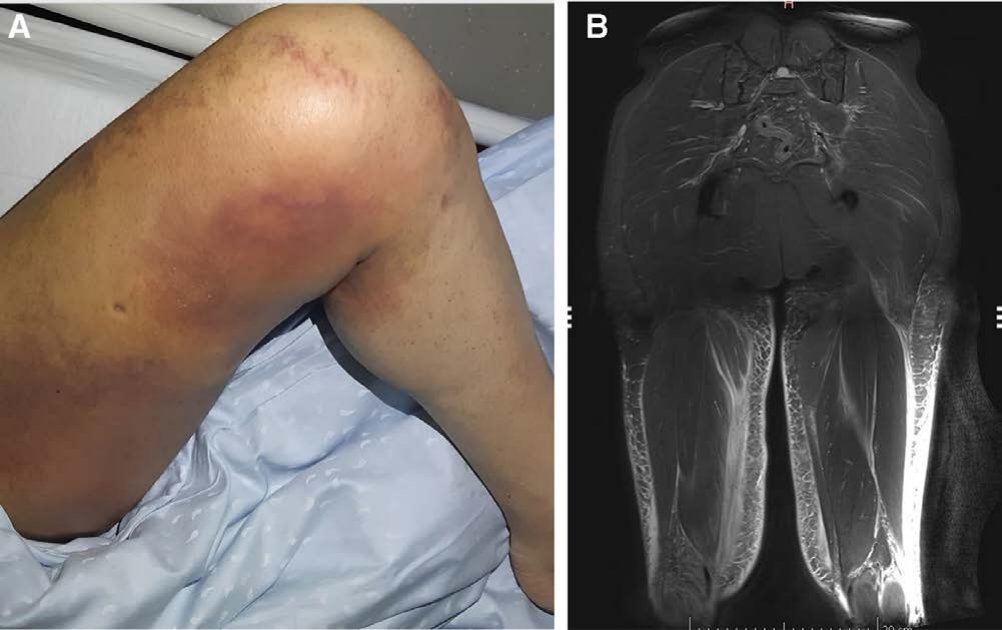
(A) Picture of the lower limbs showing both edema and hematomas. (B) T1-weighted MRI without contrast showing a bilateral extensive fluid infiltration of the skin and subcutaneous tissues, extending to muscular aponeuroses. MRI = magnetic resonance imaging.
